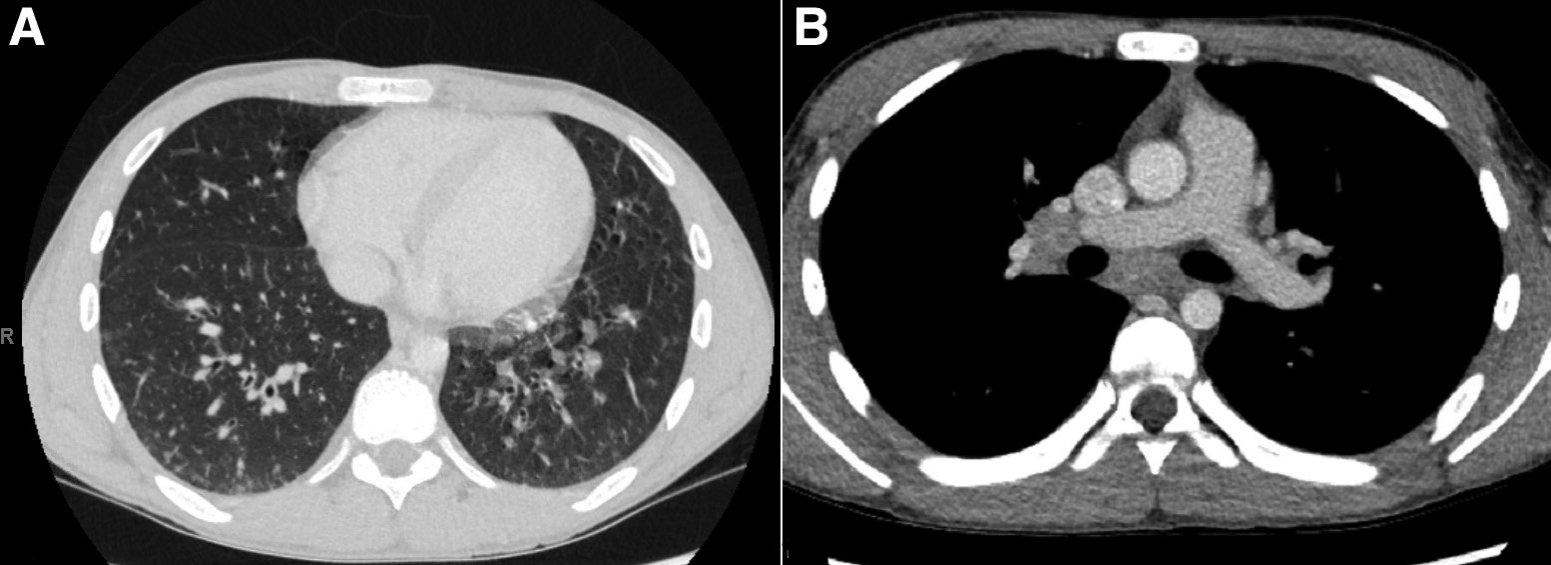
Chest computed tomography at presentation (A) Bibasilar consolidations and ground glass opacities are seen, mild bibasilar predominant bronchial wall thickening (B) Enlarged right hilar and mediastinal lymph nodes.
